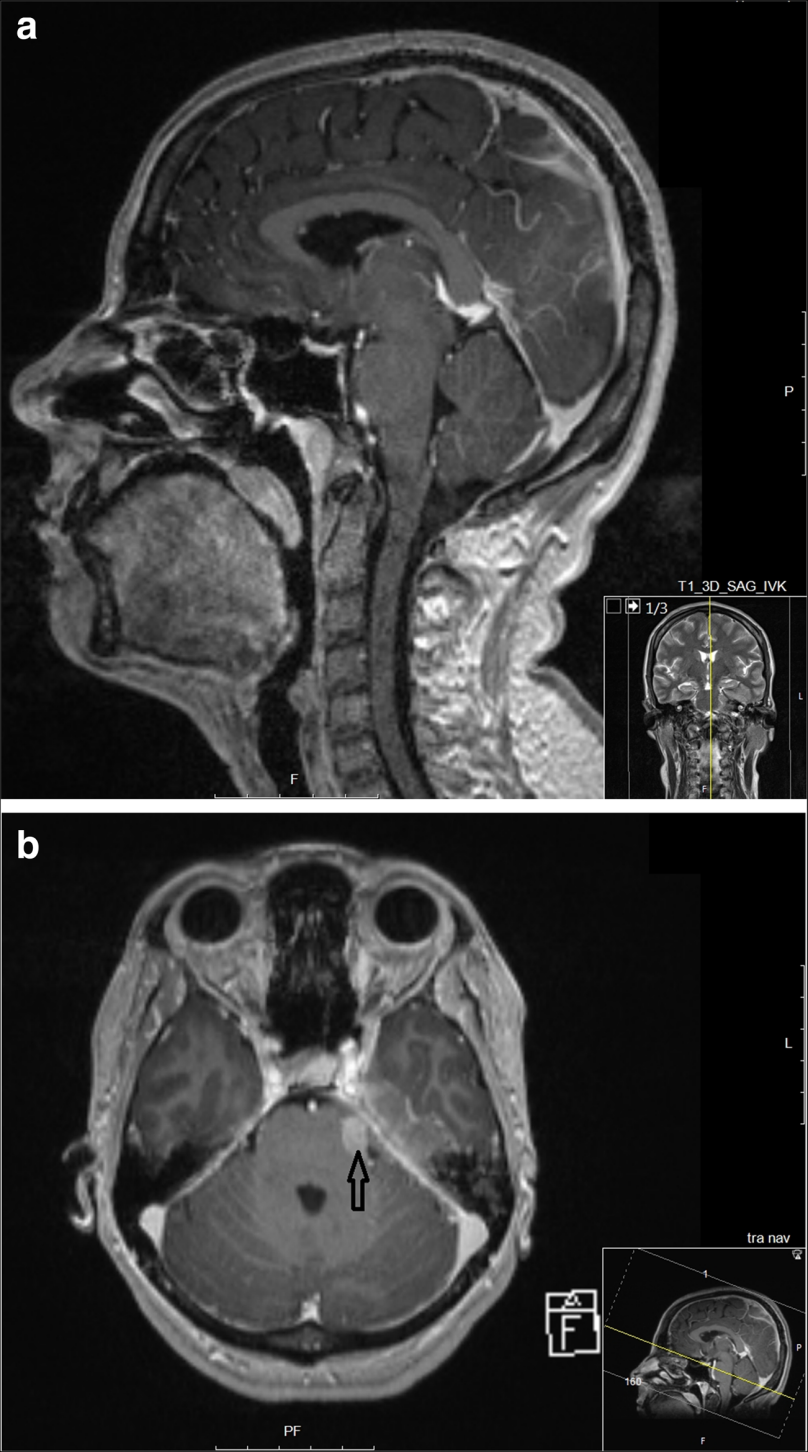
a Sagittal MRI scanning before initiation of oncological treatment. No sign of herniation was seen, and no sign of obstructive hydrocephalus. b Axial MRI scanning showing peri-pontine tumor infiltration (arrow)
